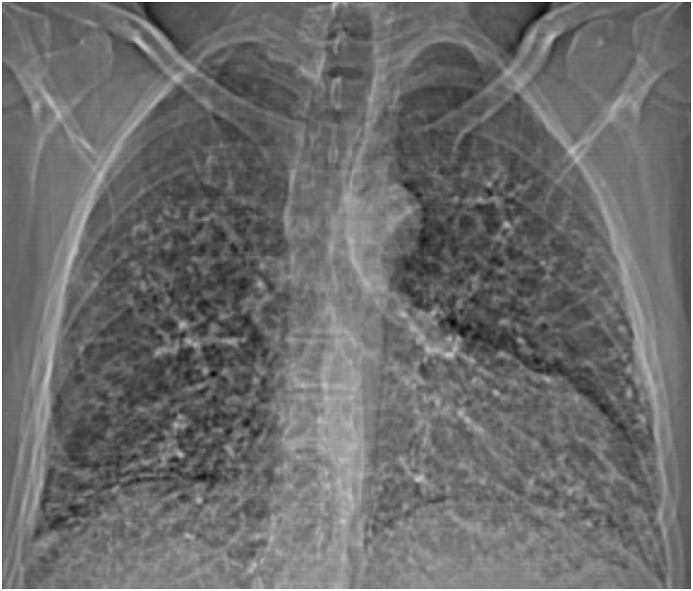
Chest x-ray demonstrating bilateral calcifications and interstitial changes on initial workup.
